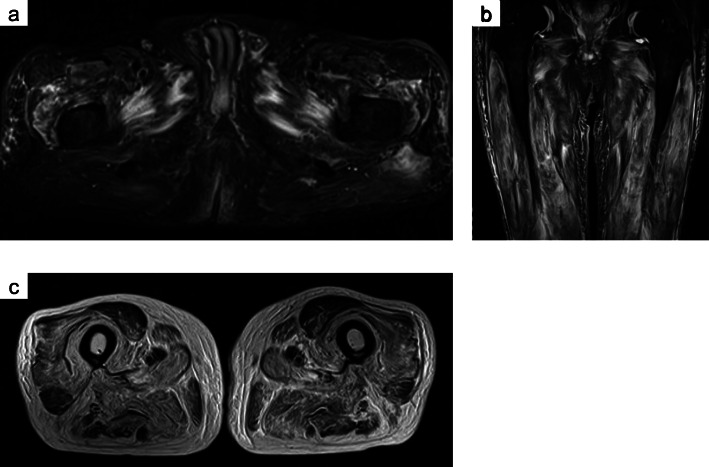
Muscle magnetic resonance imaging scan images. Axial (a) and coronal (b) short inversion time inversion recovery (STIR) images and axial T2 weighted image (c) show muscle atrophy and high intensity signals in the quadriceps femoris, obturator, and gluteus maximus muscles
